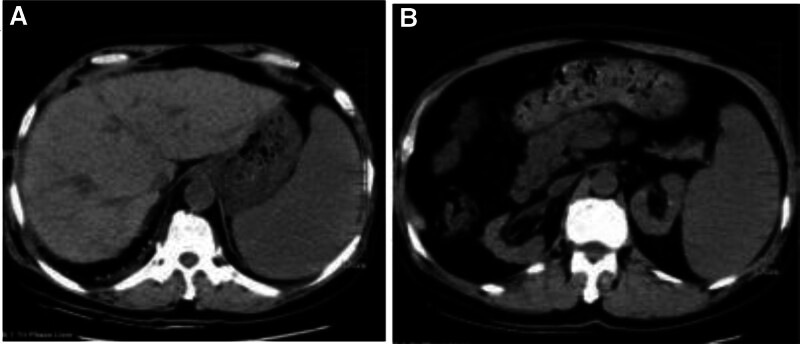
(A and B). Upper abdominal CT showed liver cirrhosis, splenomegaly, and portal hypertension. CT = computed tomography.
